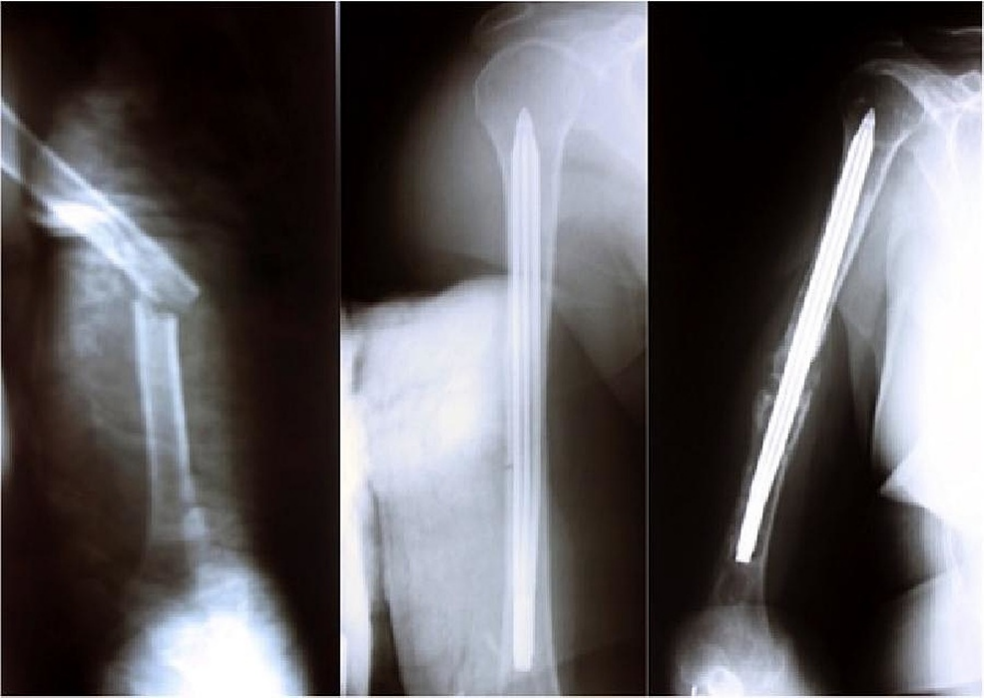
Fixation of humeral shaft fracture with retrograde expandable nail. Complete reduction was obtained in early postop radiography. However, osteolysis and nonunion developed in the distal part according to follow-up radiographs.
